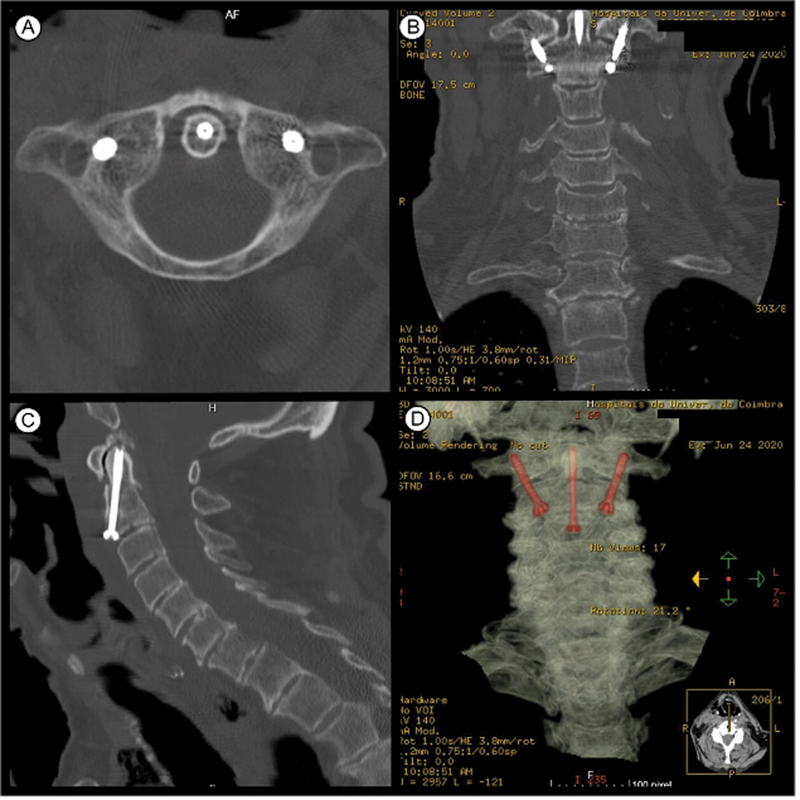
Postoperative CT scan showing correct alignment of the screws on the axial (
